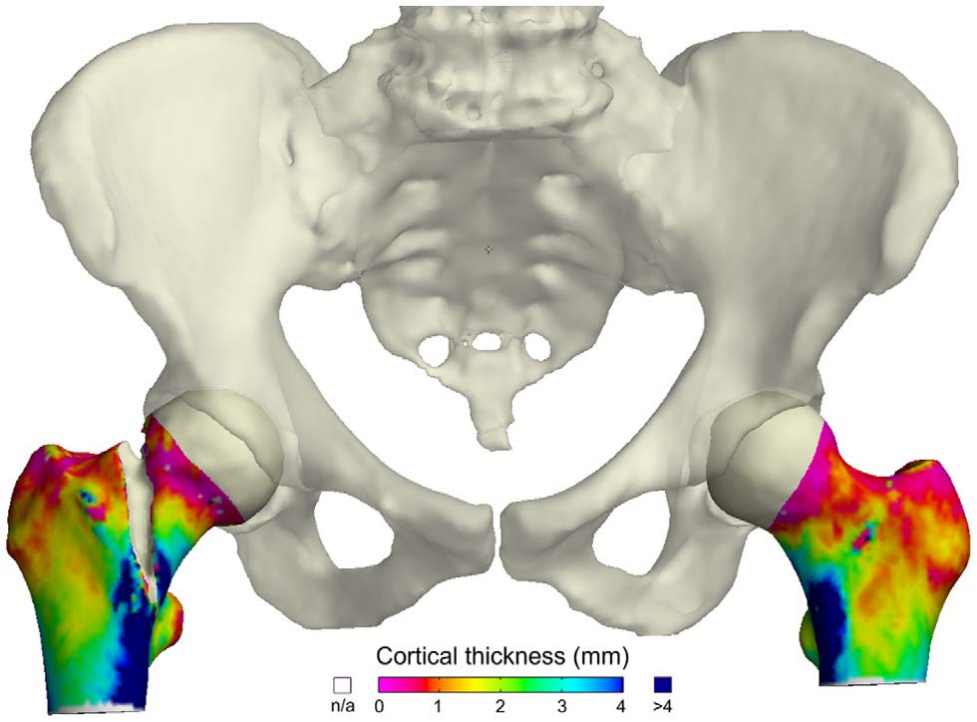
Cortical Thickness Colour Mapping using ordinary clinical CT data. Femora and pelvis from an 84-year-old osteoporotic female who sustained a fracture without falling. She felt her right hip break as she placed her right foot on a low step. Femoral neck BMD was 0.46 g/cm2, T score −3.3. From the Arthritis Research UK FEMCO study (07/H0305/61).
